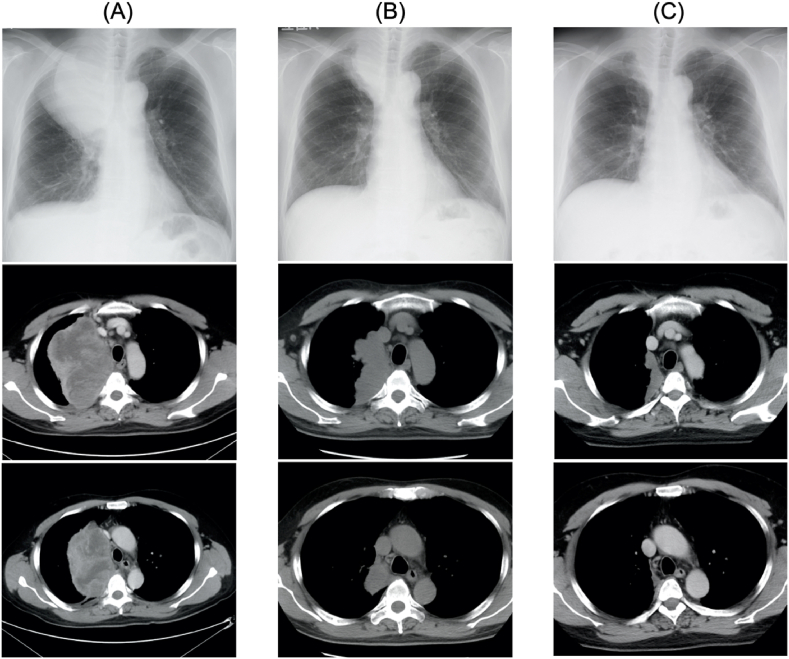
(A) Chest radiograph and chest CT images on admission. Chest radiograph image on admission shows a tumor shadow in the right upper lung field. Chest CT images reveal a huge tumor in the right upper lobe, invading the mediastinum and thoracic wall, and right small pleural effusion. The density of the tumor is irregular. (B) Five months after the initiation of therapy, the chest radiograph shows a noticeable reduction of the tumor shadow in the right upper lung field. Chest CT shows evident reduction of the tumor size in the right upper lobe, no significant mediastinal lymphadenopathy, and no pleural effusion. (C) Two years after the initiation of therapy: tumor shrinkage persists.
